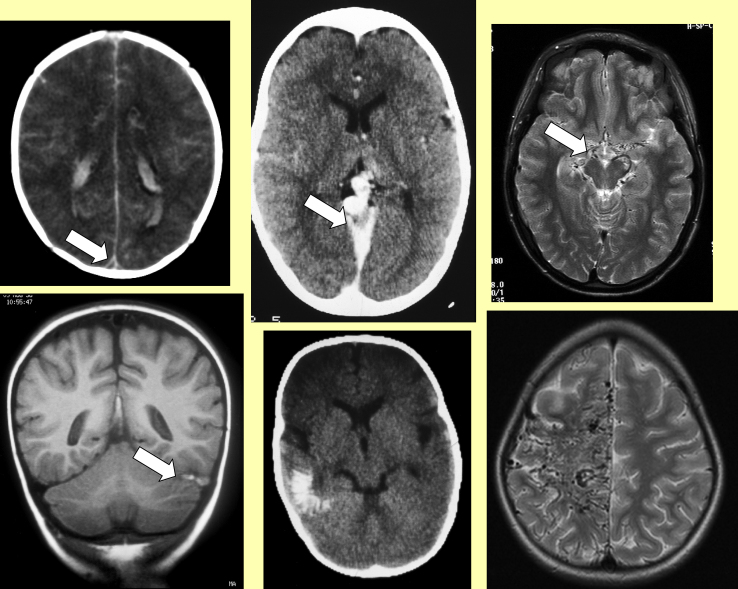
Vascular abnormalities seen on parenchymal imaging. Left: Venous thrombosis in the sagittal sinus (Top: empty delta sign on contrast CT scan; Bottom: thrombosis in the transverse sinus on MRI) Middle: Other vascular abnormalities (Top: contrast CT showing a vein of Galen malformation in a child with proptosis; ‘tramline’ calcification on CT in a child with Sturge–Weber syndrome) Bottom: Abnormal arteries seen as filling defects (Top: collaterals in moyamoya; Bottom: giant arteriovenous malformation) Right:
